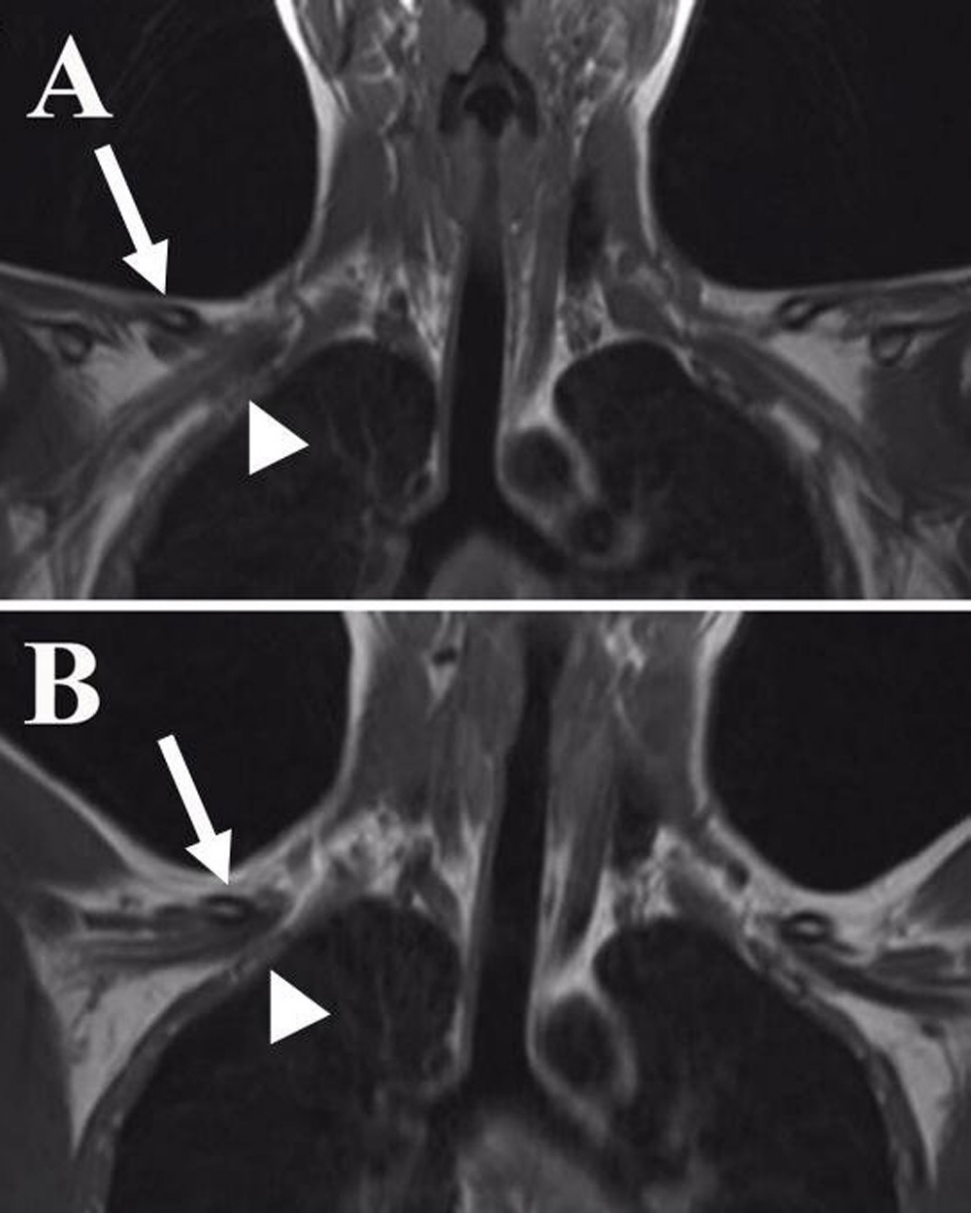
Case 1: (A-B) MRI T1-weighted coronal images showing that the space between the cervical rib (arrow) and the first rib (arrow head) diminishes upon lifting the upper arm with subsequent impingement of the brachial plexus in image B.
